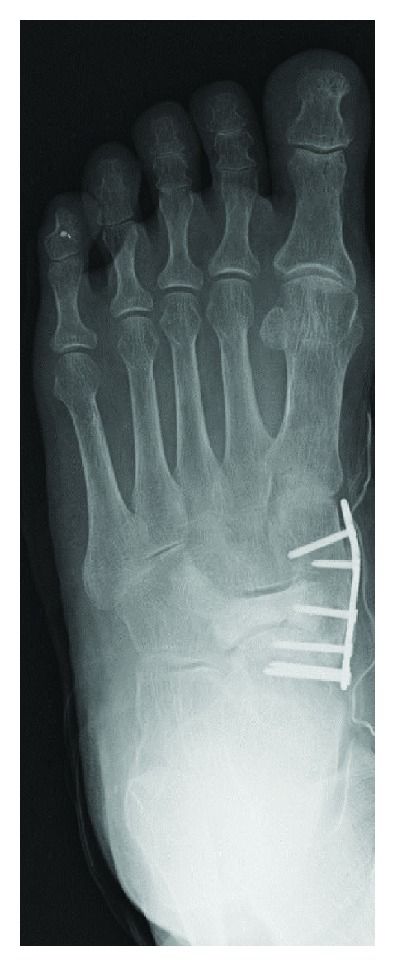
Postoperative radiograph shows fixation using LCP-DRP with 6 2.4 mm locking screws.
